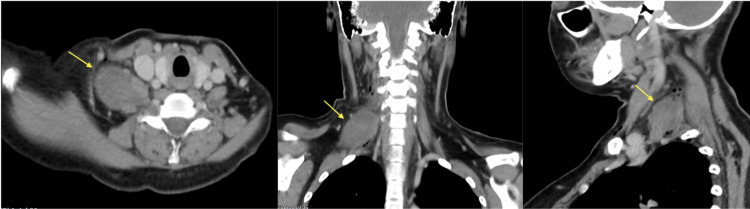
CT scan of the neck with contrast showed a fusiform, heterogeneous enhanced soft tissue lesion (yellow arrow) arising from the right C5 and C6 cervical nerve root causing the relative widening of the neural foramina fullness of the right C5/C6 and C6/C7.
